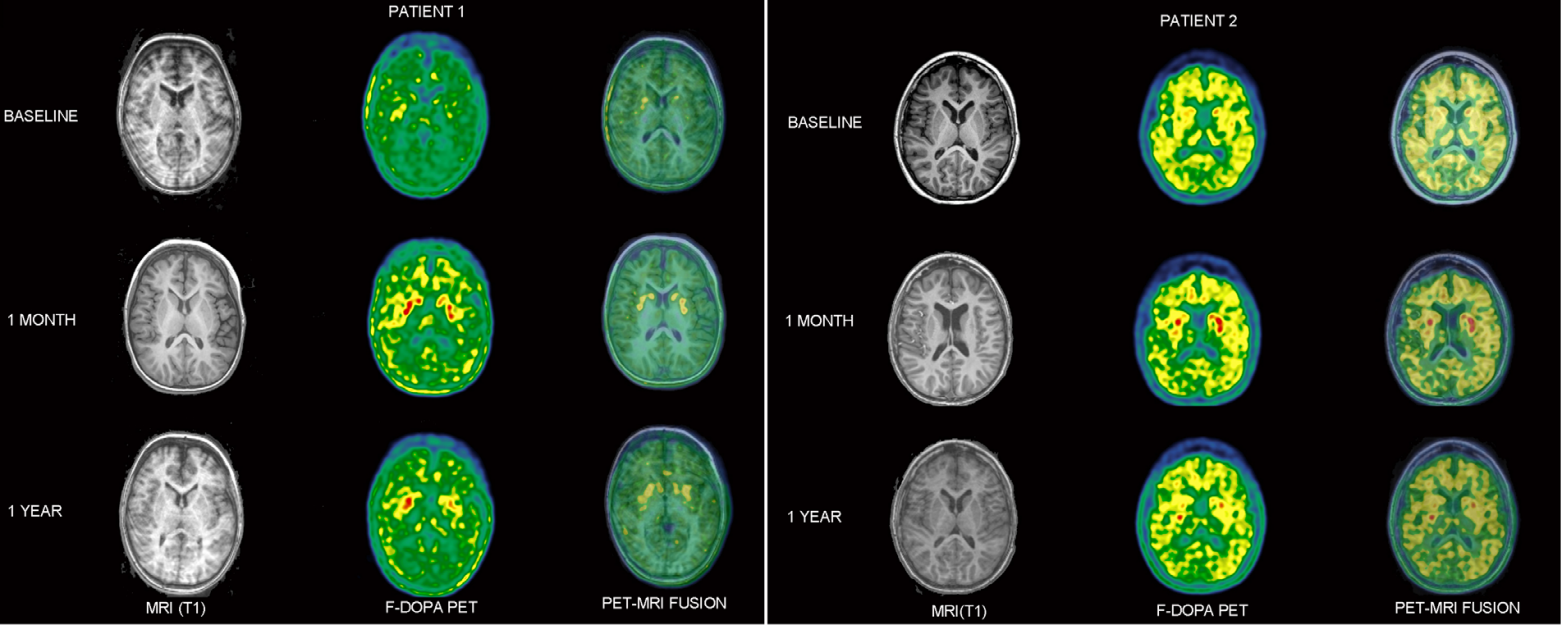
^18^F‐DOPA PET and MRI images at baseline, 1 month, and 1 year after gene delivery. The color palette was centered on the maximum signal observed in the cortical gray matter, to allow visual comparison between time points and patients. ^18^F‐DOPA PET scan visualizes the capacity of the neurons of the putamen to durably take up ^18^F‐DOPA, which implies an efficient decarboxylation of this amino acid. Increased fixation is observed 1 month and 1 year after gene delivery. ^18^F‐DOPA, l‐6‐[^18^F] fluoro‐3, 4‐dihydroxyphenylalanine; PET, positron emission tomography; MRI, magnetic resonance imaging.
